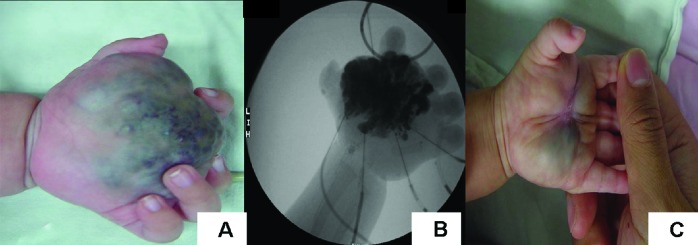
The patient in these images was 10 months old when treated. When the patient was born, a tumor was discovered on the left hand, which gradually increased in size. (A) The patient was diagnosed with venous malformation. (B) Percutaneous interventional sclerosing therapy was guided by MRI. During surgery 6 mg bleomycin, 3 ml iohexol and 3 ml ultra-liquefied lipiodol were used. The patient was treated with interventional sclerosing therapy a total of three times. (C) The patient was reviewed 6 months after surgery and the size of the tumor had reduced.
